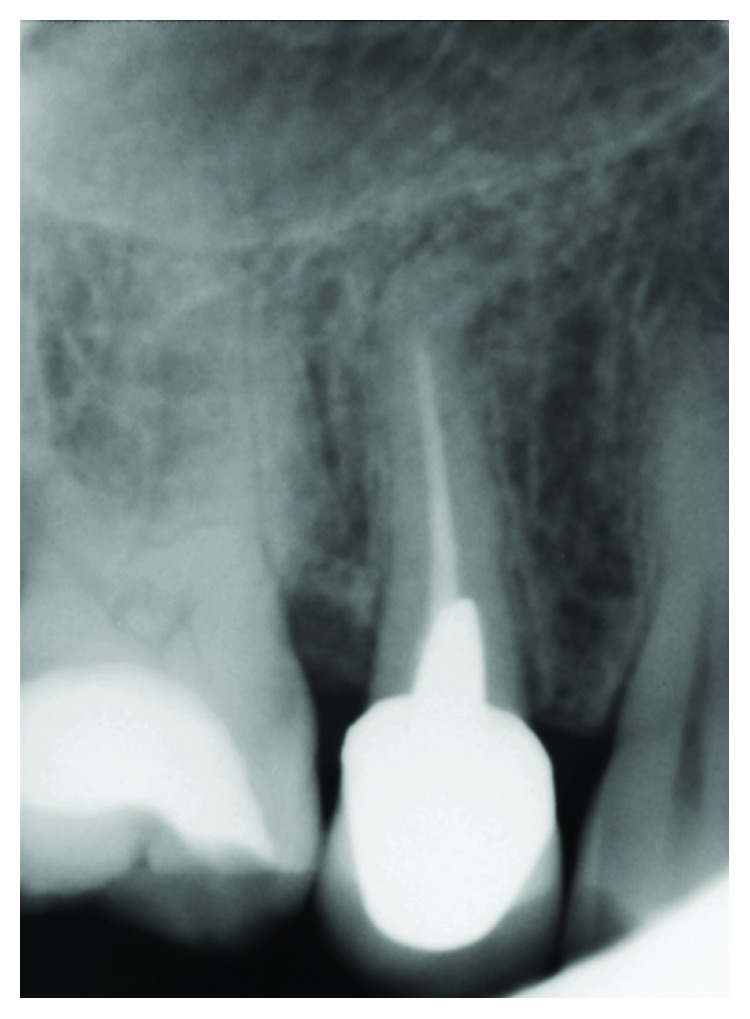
Periapical radiograph showing previous endodontic and prosthetic treatments on upper second premolar.
